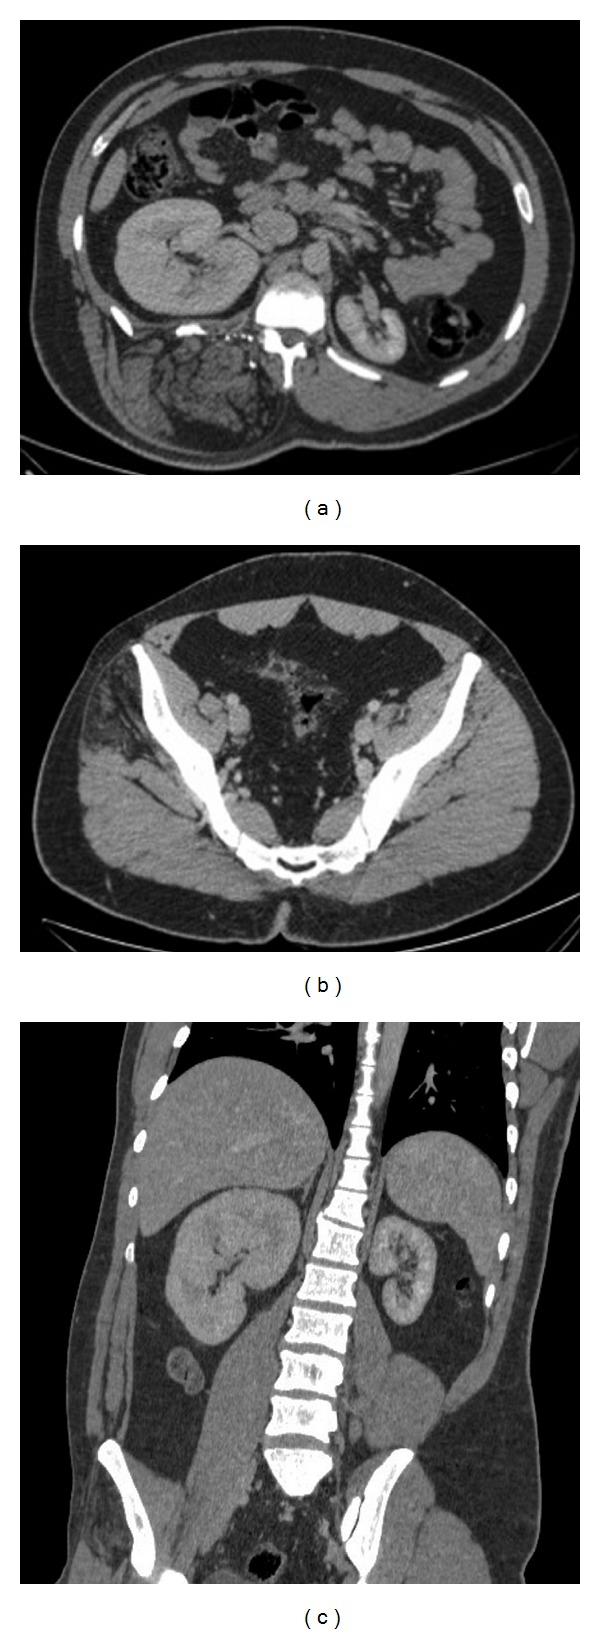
A 21-year-old man with Proteus syndrome presenting asymmetric lower limbs and epidermal nevus. CT of the abdomen showed tissue with a fat density infiltrating the right paraspinal musculature, with increased local volume extending from T7 to L5, in addition to an enlargement of the right kidney (a). The scan also noted fatty replacement in right gluteal muscles (b). Coronal CT showed asymmetry of the kidneys (c).
